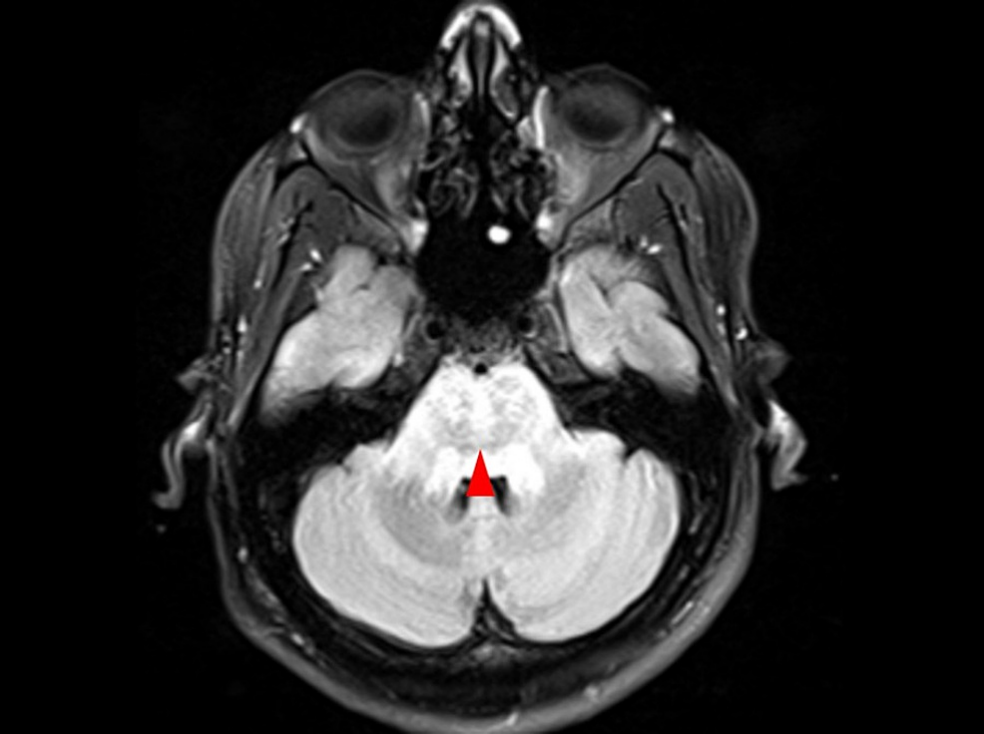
Axial MRI FLAIR sequence showing a hyperintense signal mainly involving the pons (arrowhead). MRI: magnetic resonance imaging; FLAIR: fluid-attenuated inversion recovery
